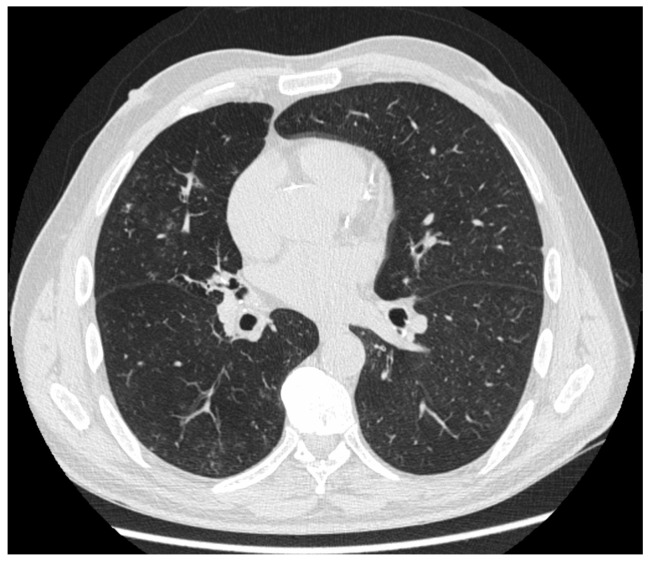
Chest HRCT, axial planes, patient affected by chronic aspiration pneumonia. CT scan shows centrilobular micronodules and GGO due to the surrounding parenchyma inflammation, and occlusive aspiration of the medium lobe bronchus, with relative pulmonary atelectasis.
